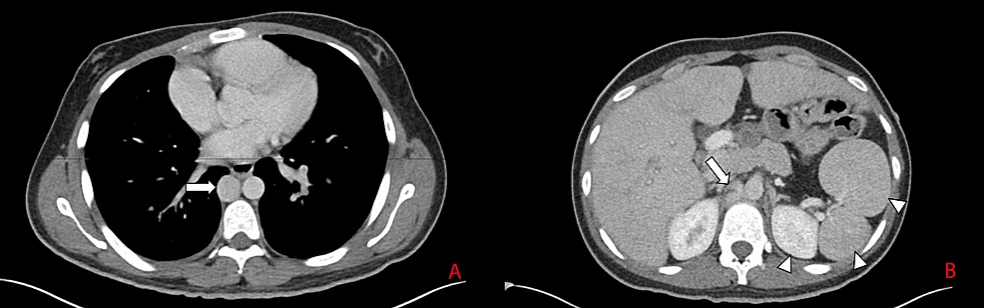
(A) Axial view of CTPA in the venous phase showing the enlarged azygos vein (arrow) coursing alongside the descending aorta. (B) Axial view of CT scan of the abdomen with IV contrast demonstrating multiple spleens (arrow heads). Also note the anastomosis between the IVC and the azygos vein (arrow). CTPA: computed tomography pulmonary angiogram, CT: computed tomography, IV: intravenous, IVC: inferior vena cava.
